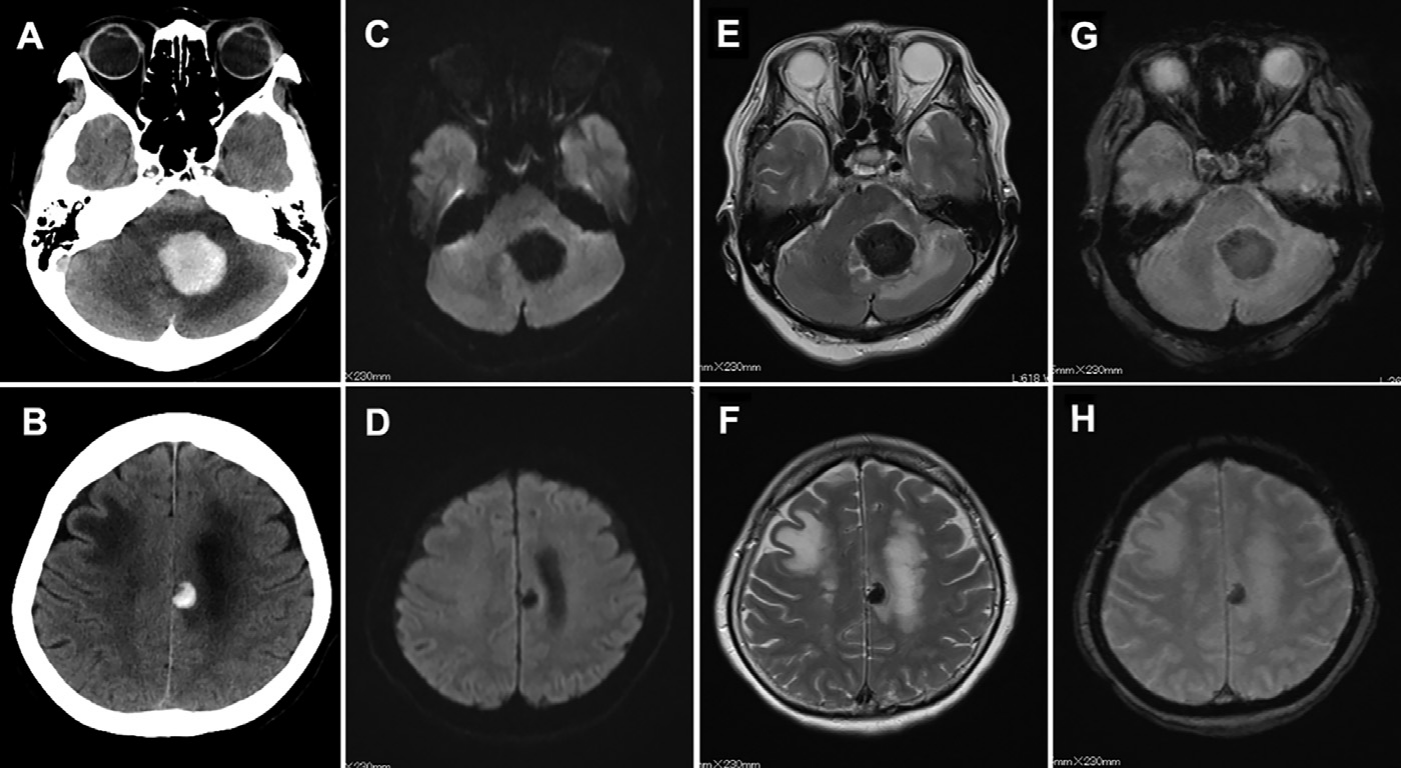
Computed tomography image showing striking high-density lesions in the left cerebellum (A) and left frontal lobe, (B) with slight perifocal edema and edematous change in the right frontal lobe. Diffusion (C, D), T2 (E, F), and T2* (G, H) -weighted magnetic resonance imaging presented homogenous low intensity lesions in the left cerebellum and left frontal lobe, respectively.
